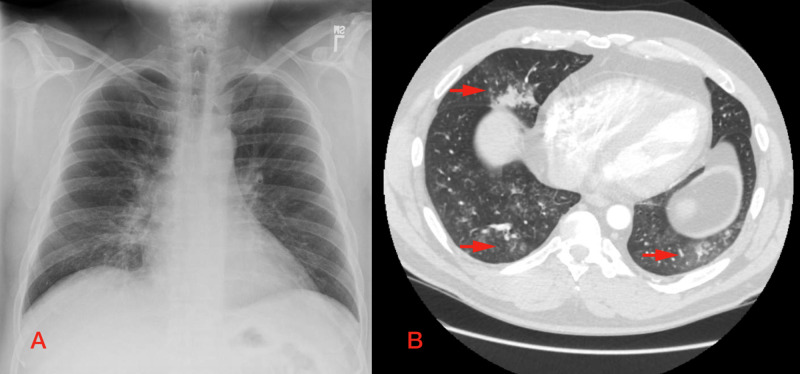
Imaging on presentation to the hospital A: initial chest X-ray demonstrates evidence of a right middle lobe infiltrate; B: CT pulmonary angiogram after initial chest X-ray shows evidence of bilateral ground-glass and nodular opacities (arrows), consistent with hypersensitive pneumonia CT: computed tomography
